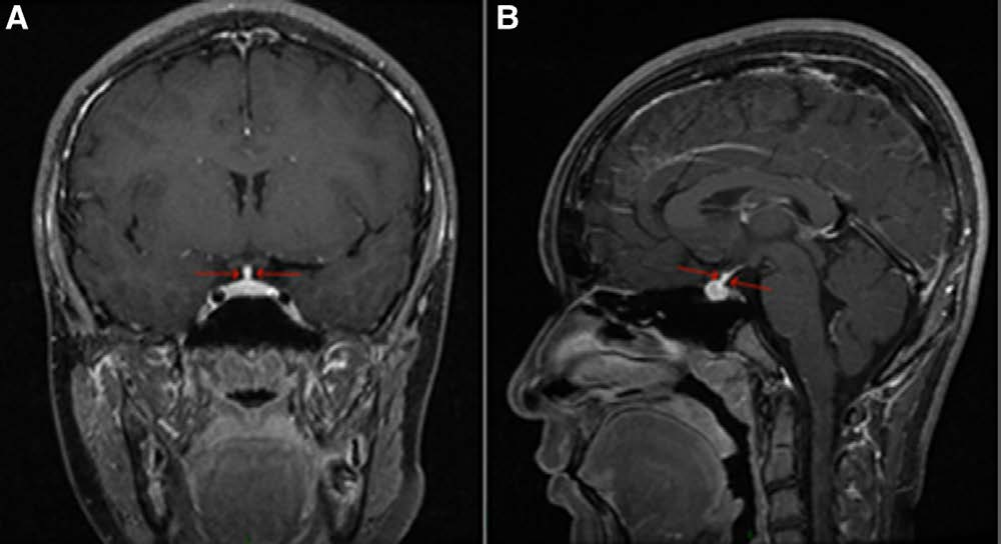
Thickening of the pituitary stalk: MRI direct dynamic enhancement scan of the pituitary gland (A: coronal, B: sagittal): the pituitary gland has an elevated diameter of approximately 6 mm (sagittal median level) and shows obvious uniform enhancement after enhancement; the pituitary stalk is centered, and the transverse diameter is thickened by approximately 3.3 mm. MRI = magnetic resonance imaging.
